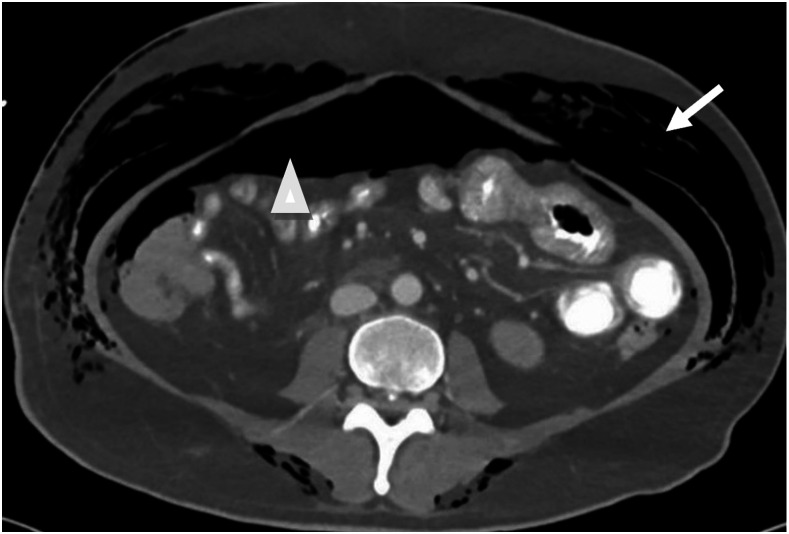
Computed tomography (CT) scan of the abdomen showing extensive surgical emphysema (arrow) and pneumoperitoneum (arrowhead). No free fluid was noted. No signs of peritoneal or mesenteric inflammation were observed.
